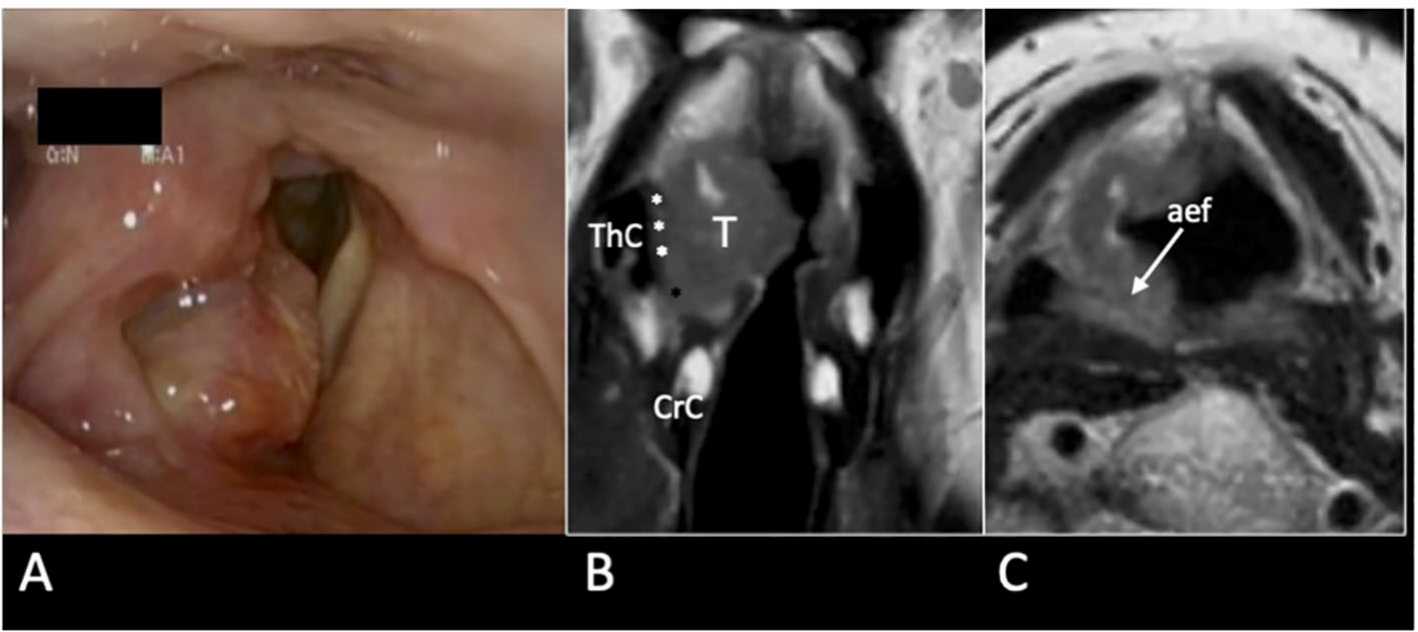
cT3 supraglottic tumour with superior paraglottic space (PGS) involvement. (A) Videoendoscopy shows a swelling of the right false vocal cord, an enlargement of the aryepiglottic fold, and a suspected transglottic extension. MRI T2-weighted sequence on coronal plane (B) demonstrates a right transglottic lesion (T) that mainly involves the supraglottic level where the tumour massively infiltrates the superior PGS (white asterisks) with a “weight” effect on the arytenoid. Inferiorly, the tumor involves the PGS at the glottic level (black asterisk). The thyroid (ThC) and the cricoid (CrC) cartilages do not show any sign of invasion. On axial plane (C) submucosal involvement of the right aryepiglottic fold is pointed (white arrow).
